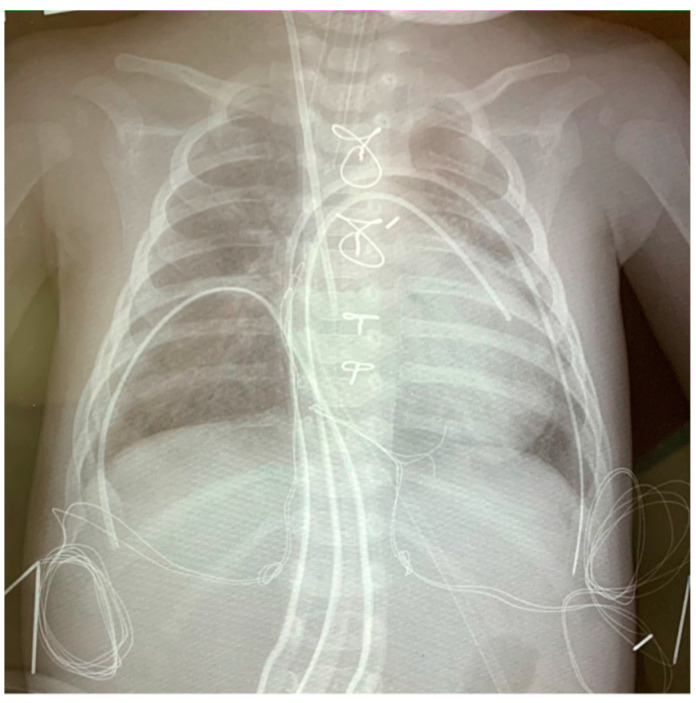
Chest X-ray. The sternum was sutured with a wire ligature, with the formation of an eight-shaped suture on the handle and on the body of the sternum. The pleural cavities and the retrosternal space with pericardium were drained separately.
